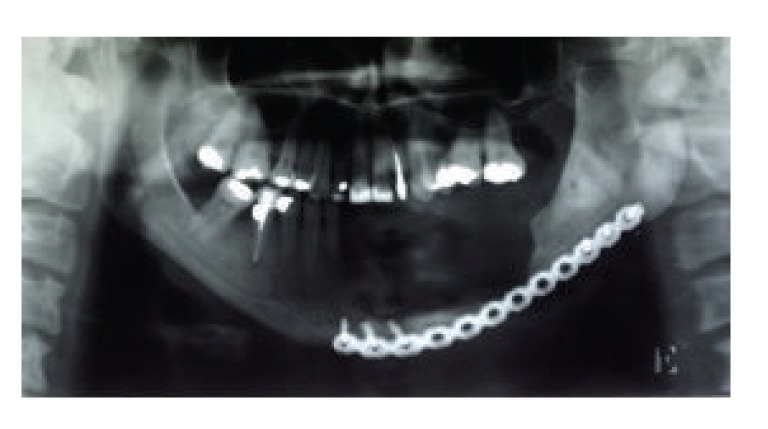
Postoperative X-ray showing surgical margins and the continuity of the base of the mandible.
